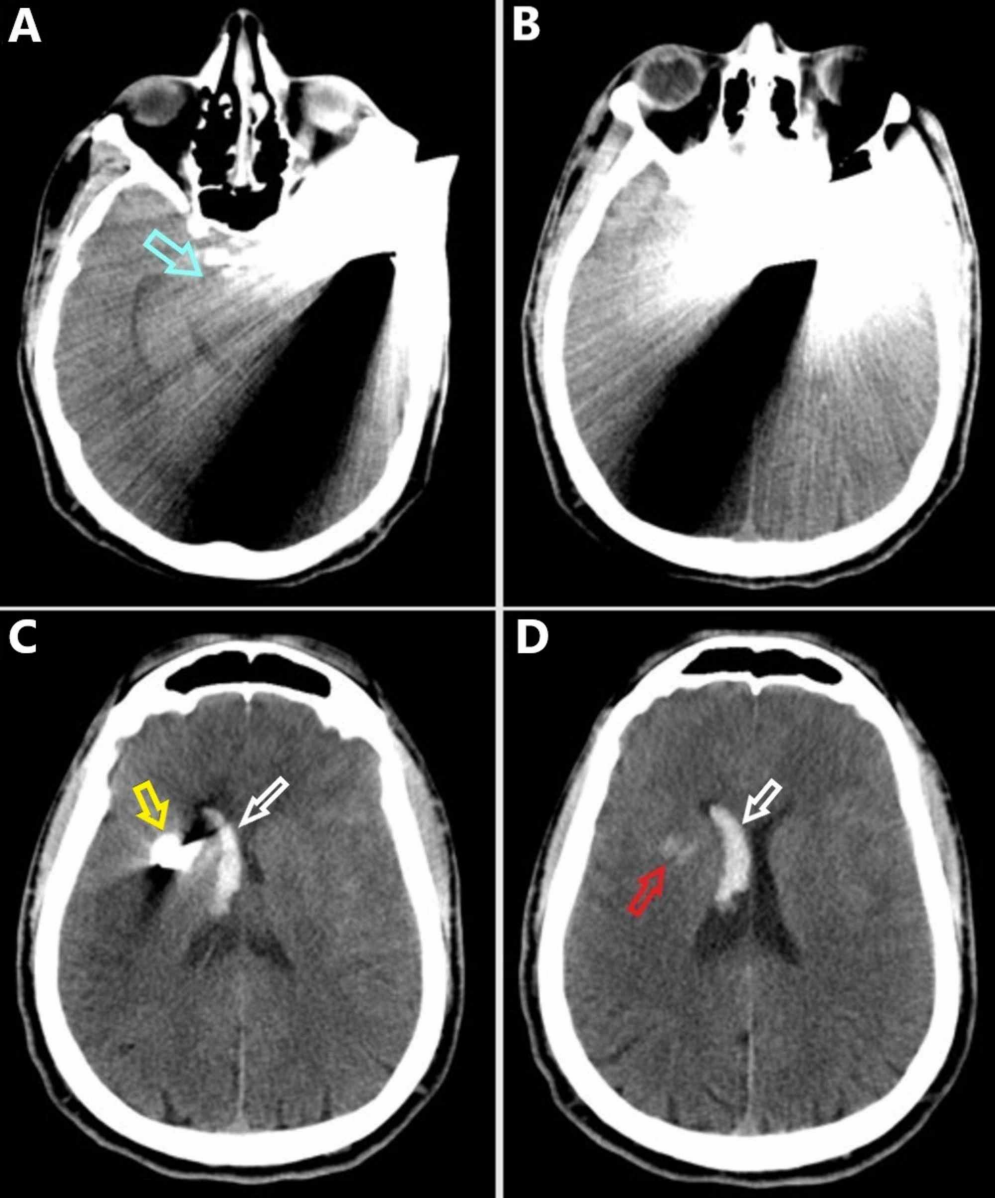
Noncontrasted preoperative CT scan of the head in the axial plane progressing from inferior (A) to superior (D). (A) The knife penetrates the calvarium in the left temporal region. Note the midbrain is in close proximity to the injury tract (blue arrow). (B) The knife begins to penetrate across midline. (C) The tip of the knife is evident in the right hemisphere (yellow arrow). Note the intraventricular hemorrhage in the right lateral ventricle frontal horn and body (white arrow). (D) There is a small intraparenchymal hemorrhage in the right basal ganglia (red arrow). Intraventricular hemorrhage is evident within the right lateral ventricle frontal horn and body (white arrow).
